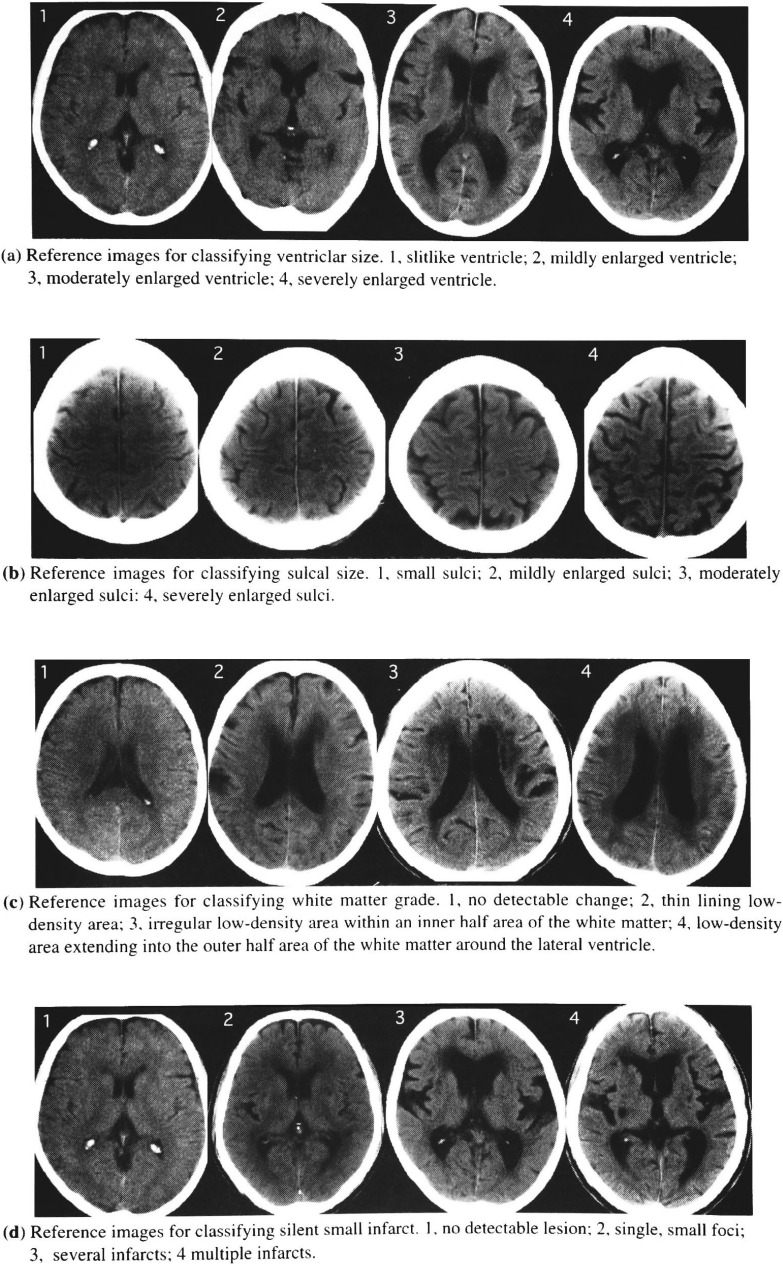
Predefined visual standards for classifying changes in the ageing brain detected on CT.
